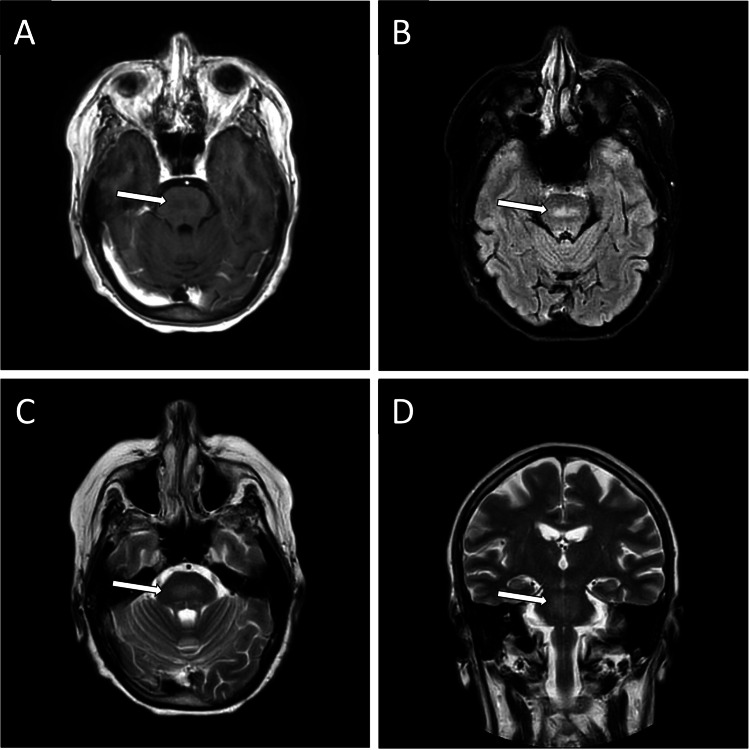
Cranial MRI (A) Central pontine T1W hypointense lesion, (B) and (C) FLAIR and T2W sequences showing central pontine hyperintense areas, and (D) T2W coronal sequence with pontine hyperintensity. T1W: T1-weighted image; FLAIR: Fluid-attenuated inversion recovery; T2W: T2-weighted image.
